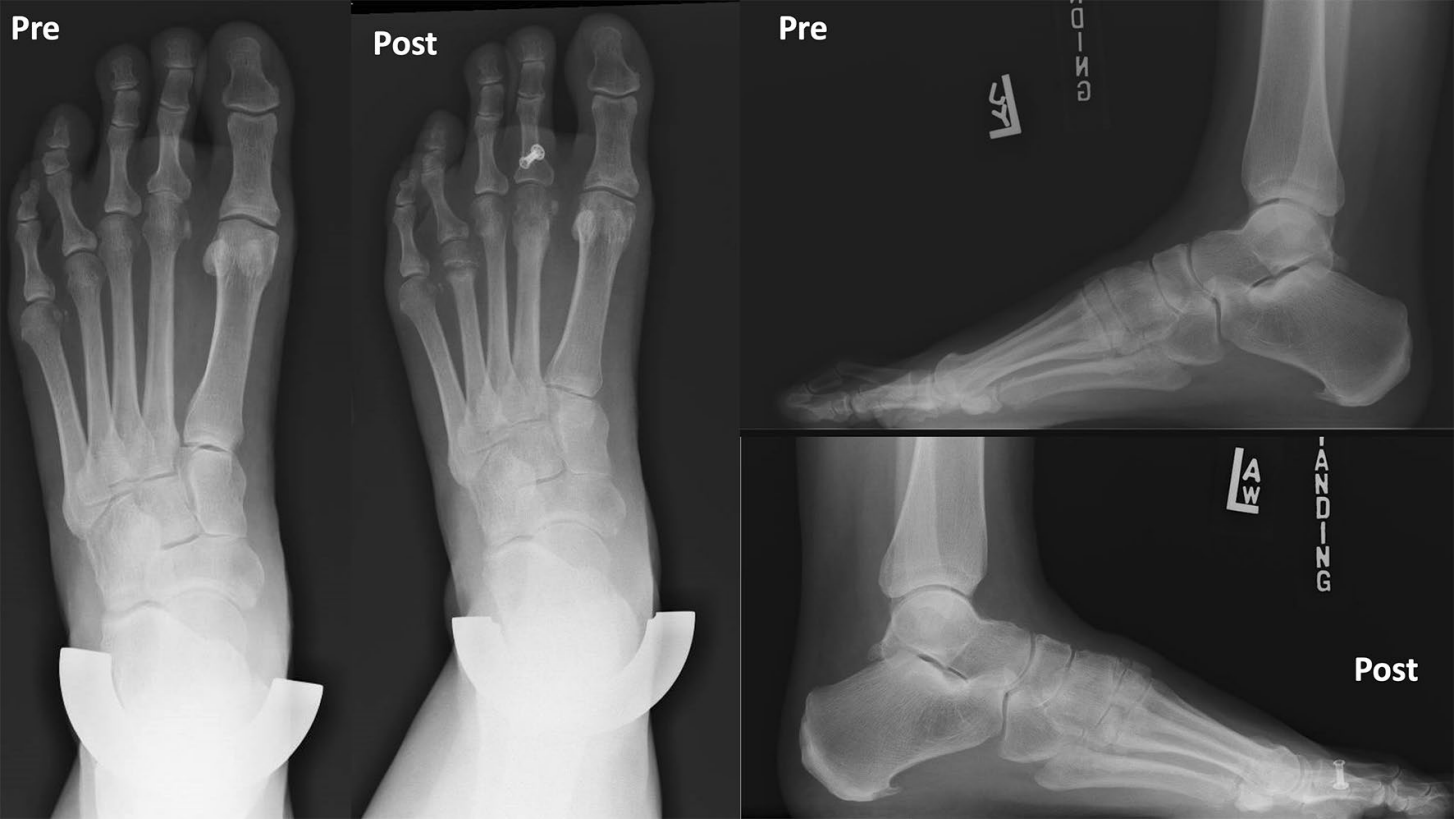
Conventional weight bearing radiographic anteroposterior and lateral views of patient’s foot, demonstrating preoperative lesser 2nd toe deformity with subluxated 2nd metatarsophalangeal joint (MTPJ), and postoperative improved alignment after 6 weeks
